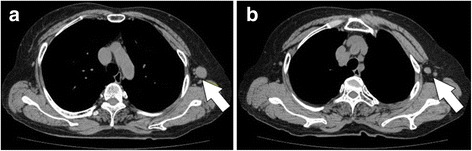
CT image findings: A systemic search using computed tomography (CT) showed multiple lymphadenopathy only in the *left axilla*, with no other systemic lymphadenopathy or mass-like lesions suspicious of a primary tumour (a, b)
